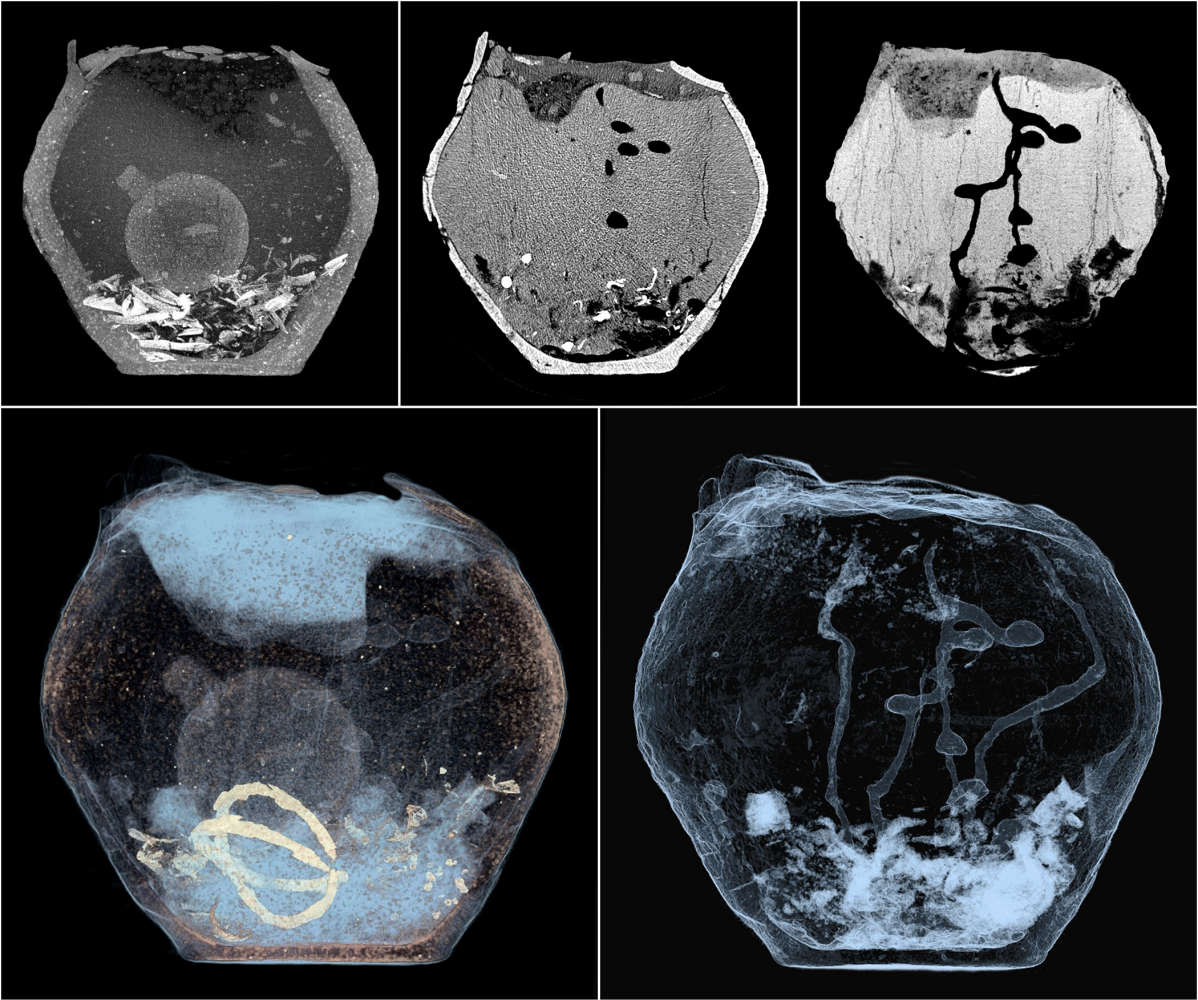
The imaging the urn B from grave 632 in order to accentuate the complexity of the elements of the fill. The MIP reconstruction shows an additional vessel placed over the burnt bones, while in the CT slices individual nest chambers of *Geotrupes stercorarius* are visible, and in the MinIP reconstruction, a part of the dung beetle nest is visualized (top row). At the bottom—the VR reconstruction, which contains all the components of the fill, including metal ornaments, and the VR reconstruction highlighting the complexity and state of preservation of the *G*. *stercorarius* nest filling up the urn.
